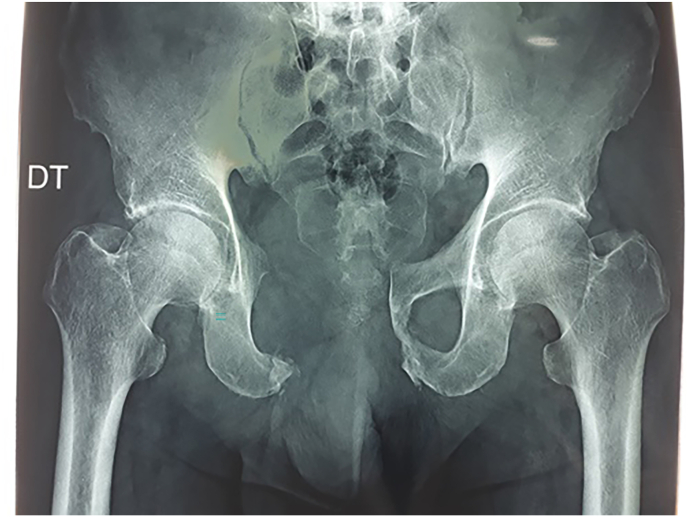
Post-operative radiograph showing complete resection of pubic tumor.
